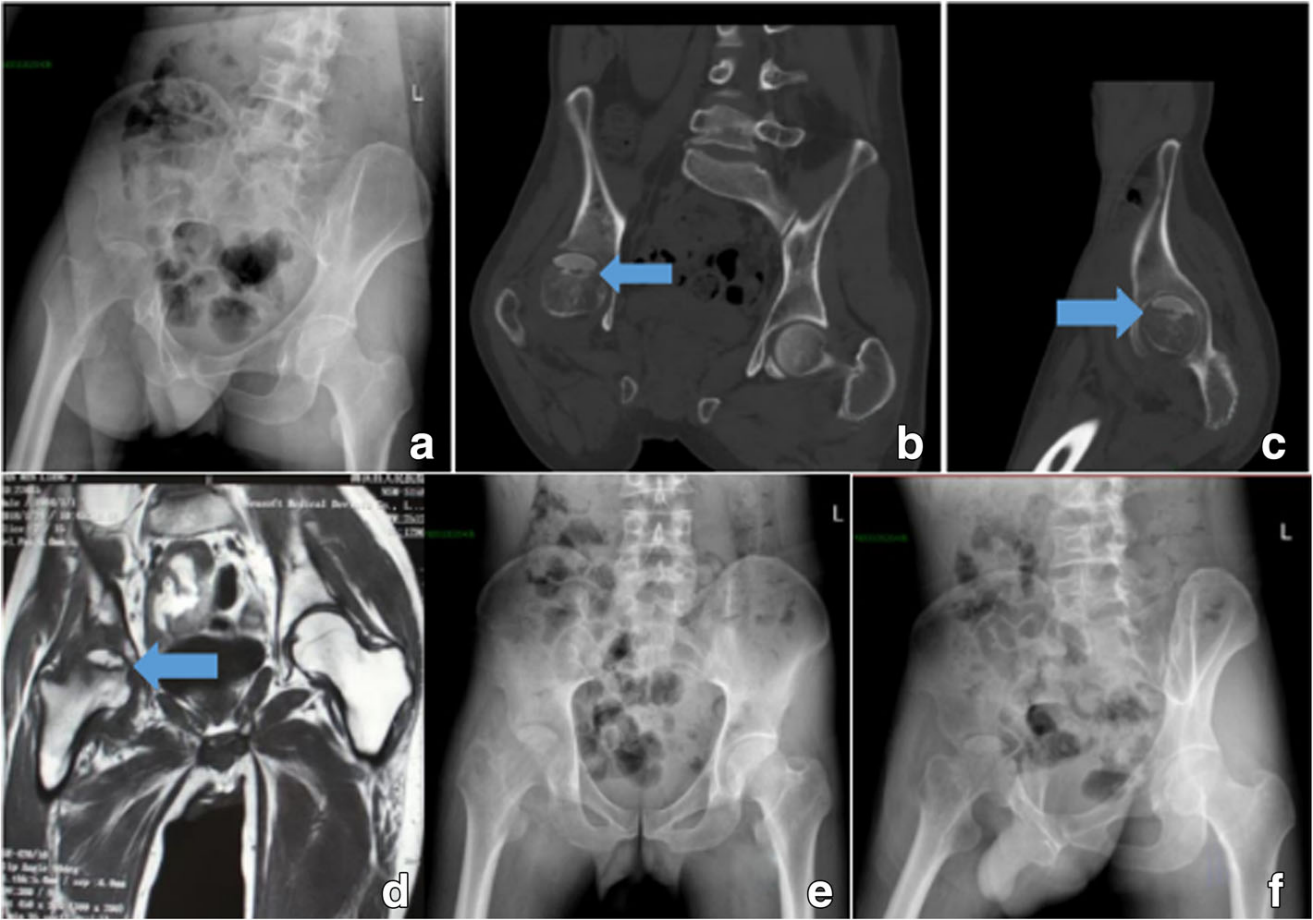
Patient 1: X-ray and computed tomography images of the pelvis showed osteonecrosis, subchondral erosion, and sclerosis (a–c). Magnetic resonance findings of the right hip (d) showed marked joint effusion and synovitis. Post-treatment X-ray images showed narrowing of the joint space and osteoporosis (e, f)
